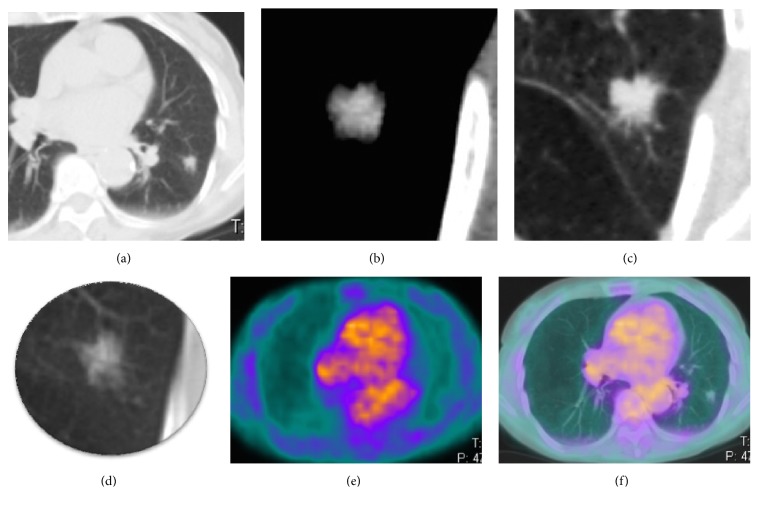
CT (a-d) and 18-FDG PET-CT (e, f) images of a 18-FDG PET false negative lung adenocarcinoma with lepidic predominant component; the solid nodule presented spiculated margins and notch sign (a and b, lung window), intense CE (97 HU) (c, mediastinal window) despite very low SUVmax (0.2) at 18-FDG PET examination (e, f); 1 year before the same nodule appeared as a ground-glass opacity on CT scan (d).
